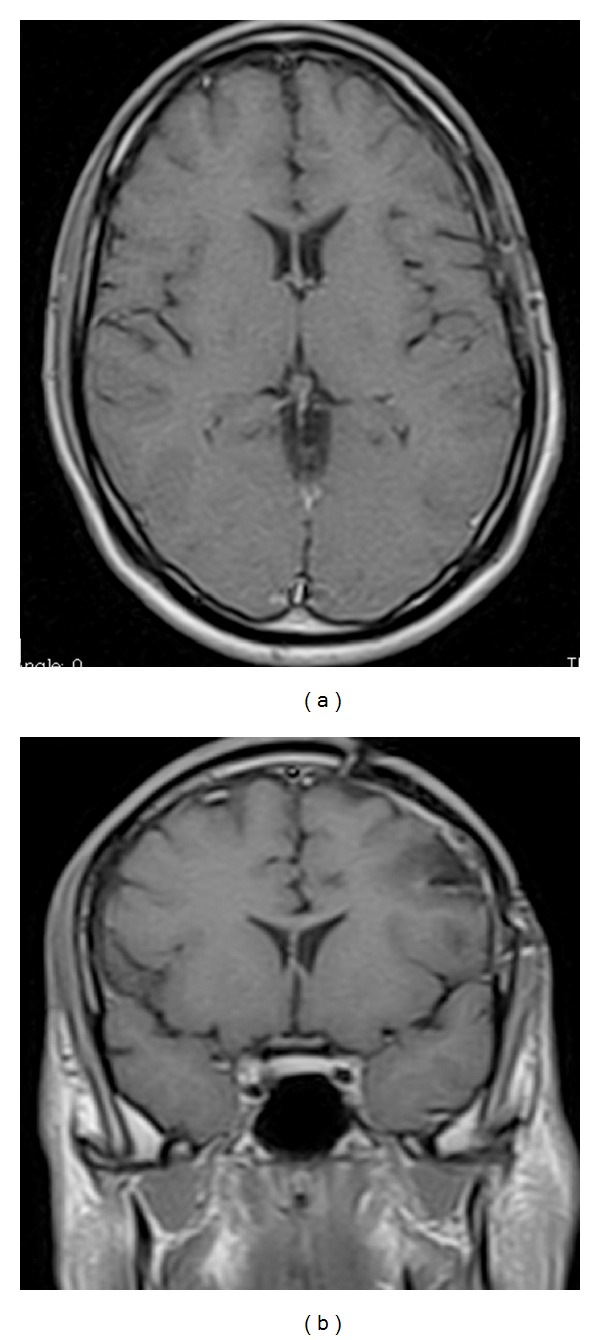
Postoperative MRI (after 4 years) in axial (a) and coronal (b) views, showing no residual tumor.
